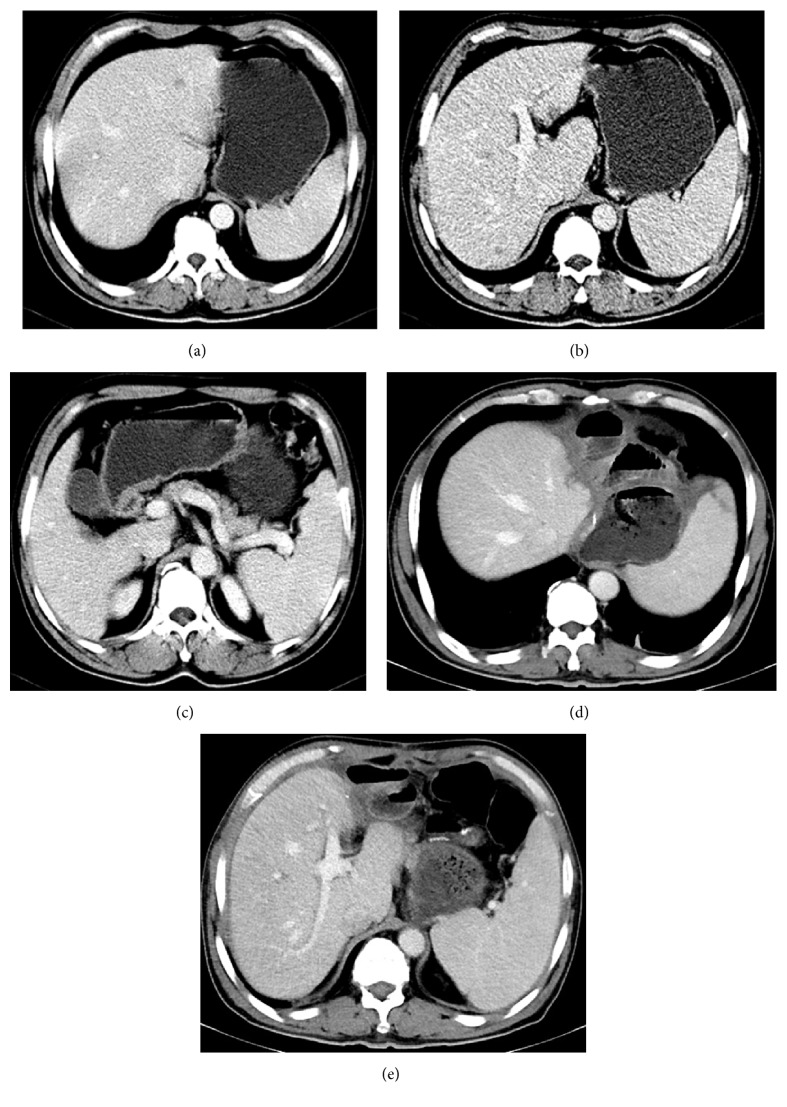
Follow-up abdominal computed tomography scans after six of EOX chemotherapy (a, b, c) and 27 months after surgery (d, e). (a) The liver metastatic nodules in liver segment 4 that had been previously observed have almost disappeared; (b) the liver metastatic lesion in the left lobe of liver that had been previously observed has evidently been reduced; (c) the gastric mass has almost disappeared; (d) postradical distal gastrectomy with Roux-en-Y with residual stomach and jejunum anastomosis and D2 lymphadenectomy combined with liver metastatic lesions resection, with no evidence of tumor recurrence 27 months after surgery; (e) no recurrence of metastatic lesions after 27 months.
